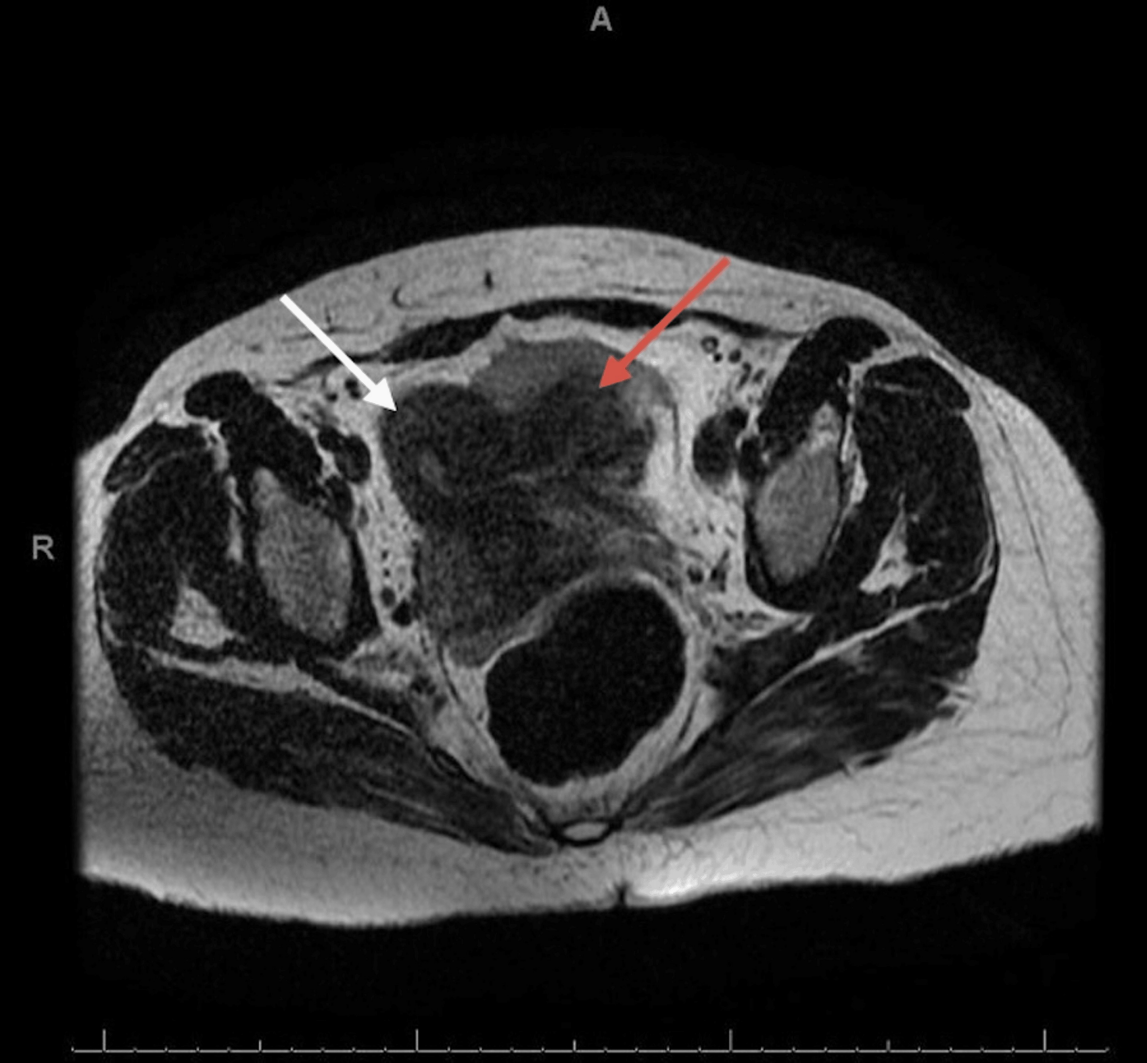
Axial image of MRI showing uterus (white arrow) with rudimentary horn (red arrow).
